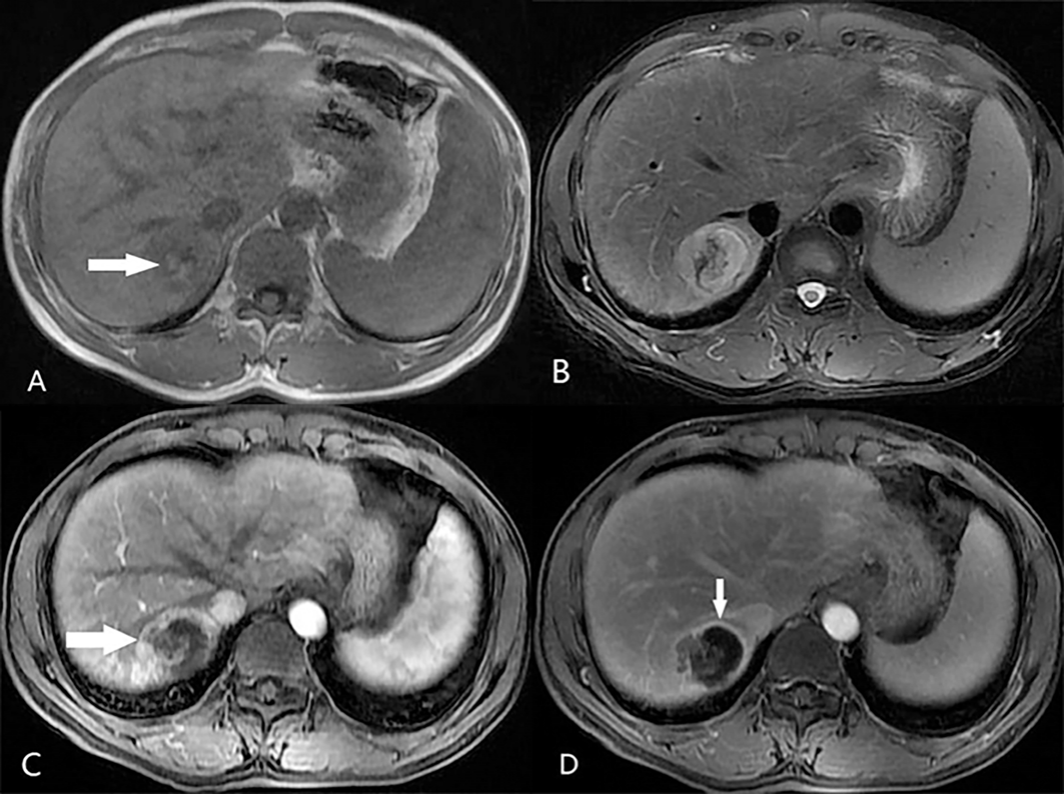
A 48-year-old male patient with hepatitis B-related liver cirrhosis and HCC is categorized as LR-M category. (A) T1-weighted image shows a 36-mm nodule with blood product in mass (arrow) in hepatic segment VIII. The nodule shows heterogeneous hyperintensity on (B) T2-weighted image. (C) The arterial phase shows peripheral enhancement (arrow) on contrast-enhanced T1-weighted imaging with the contrast agent Gd-DTPA, and (D) the delay phase shows incompletely enhancing capsule (arrows).
